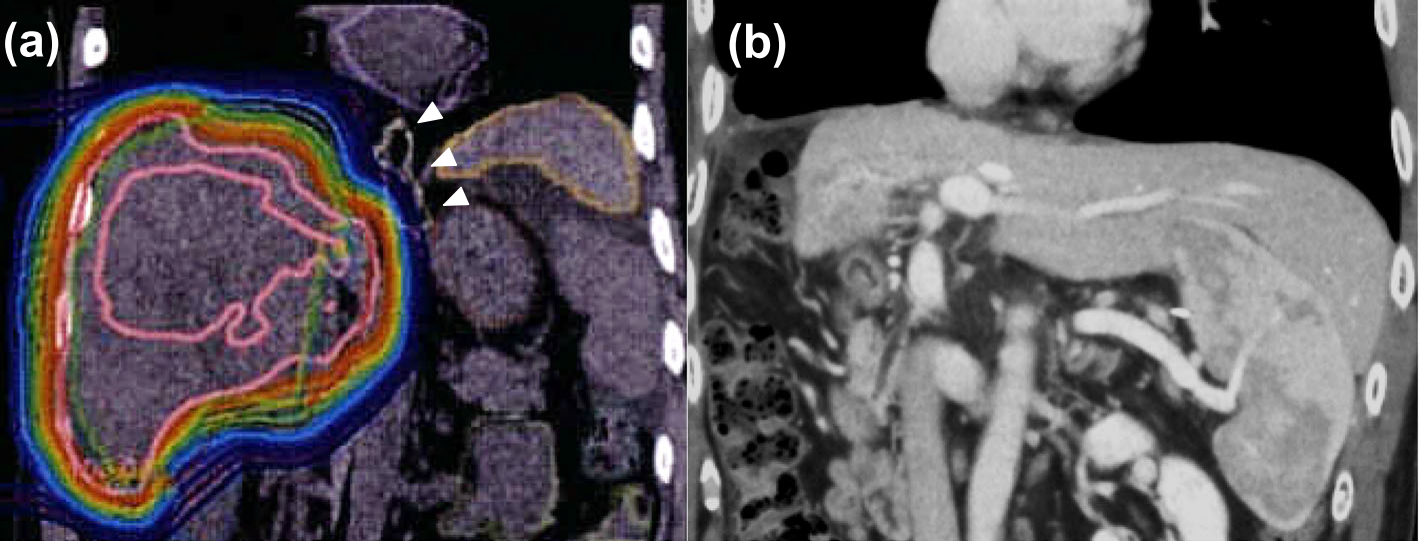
Dose distribution during proton beam therapy planning and CT findings after the therapy. a Dose distribution during proton beam therapy planning. The white arrowheads indicate the esophagus. b At 5 months after the administration of 66 GyE proton beam therapy in 10 fractions on the primary hepatocellular carcinoma with portal vein tumor thrombosis in the main trunk, CT was done, and the outcome was graded as a complete response, according to the RECIST
